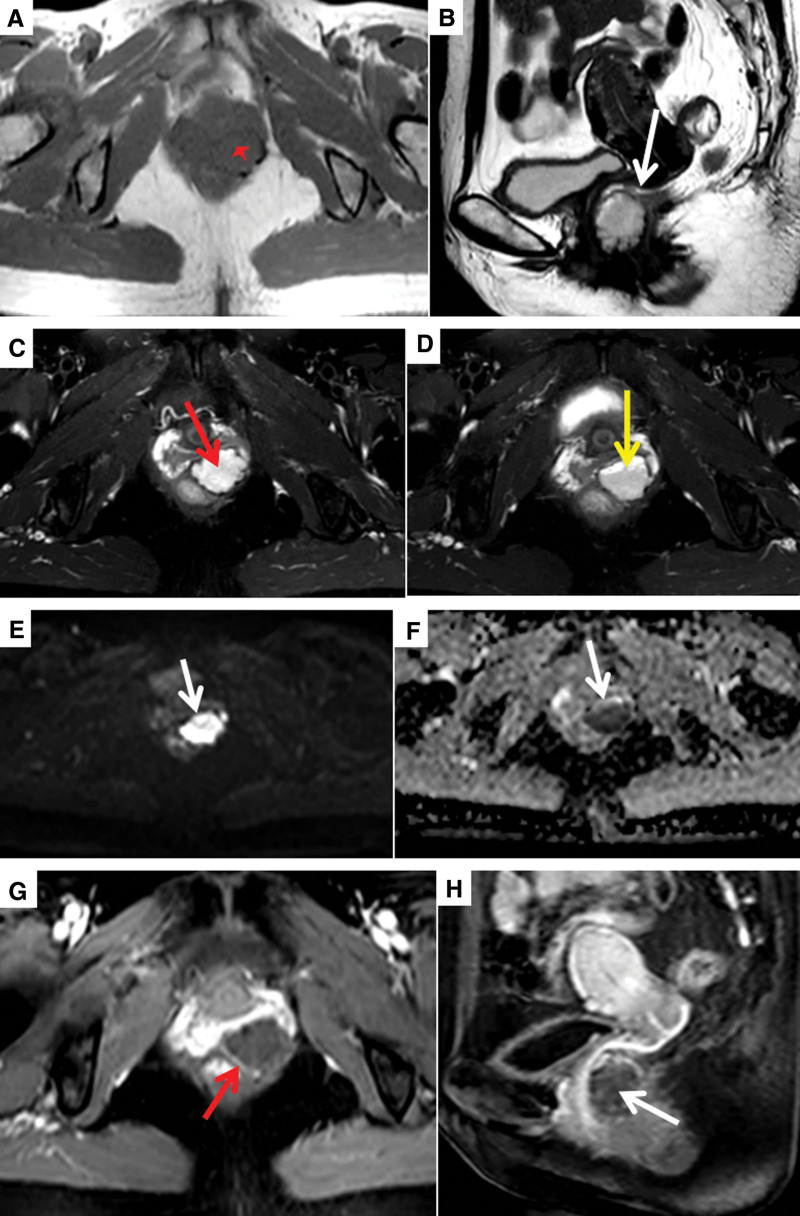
Pelvic MRI examination. (A, Axial T1WI) and (B, Sagittal T2WI), A lobulated mass (red star) can be seen in the gap between the posterior wall of the middle and lower segment of the vagina and the left anterior wall of the rectum, with a clear boundary and a size of about 30 × 22 × 28 mm, which is connected with the seromuscular layer of the left anterior wall of the rectum at an obtuse angle (white arrow), and the adjacent posterior vaginal wall is compressed and displaced forward, showing a slightly low signal on T1WI and an obvious high signal on T2WI. (C and D, Axial T2WI fat suppression sequence) shows mixed hyperintensity, with multiple fine separations (red arrow) and layered changes (liquid-liquid level, yellow arrow). (E and F) The diffusion of rectal lesions is significantly restricted (DWI high signal, ADC mixed low signal, white arrow). (G and H) After enhance, the cyst wall at the edge of the lesion showing moderate enhancement (red arrow), the septum showing mild enhancement (white arrow), and the cystic component showing no enhancement. ADC = appearant difussion coefficient, DWI = diffusion weighted imaging, MRI = magnetic resonance imaging, T1WI = T1 weighted imaging, T2WI = T2 weighted imaging.
